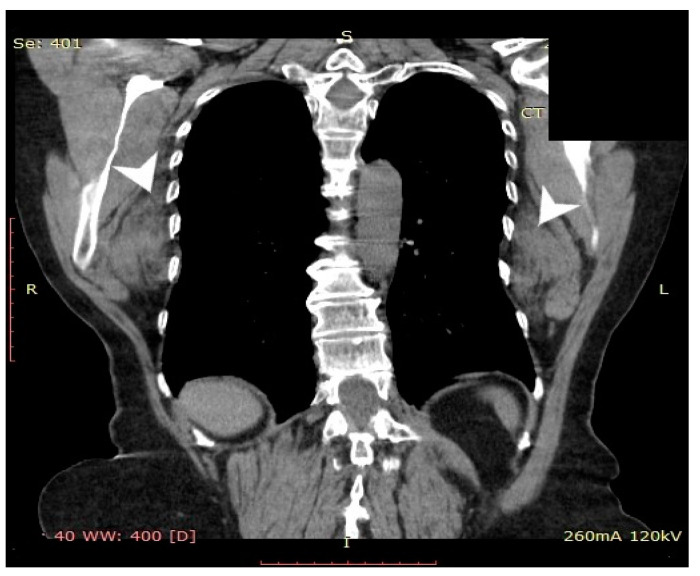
Coronal CT view of the thoracic region. The tumoral lesions are indicated by white arrows.
